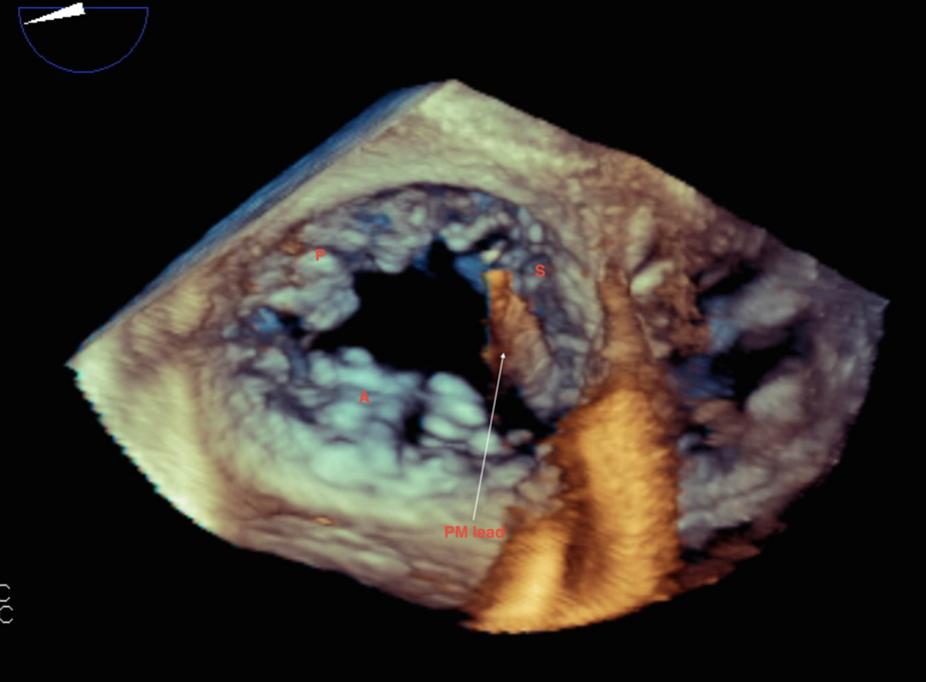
Live/real time transesophageal echocardiography. The arrow points to a pacemaker (PM) lead impinging on the septal leaflet (S) of the tricuspid valve. This caused restricted motion of the septal leaflet resulting in severe TR. Findings were confirmed at surgery. Abbreviations as in previous figures.
